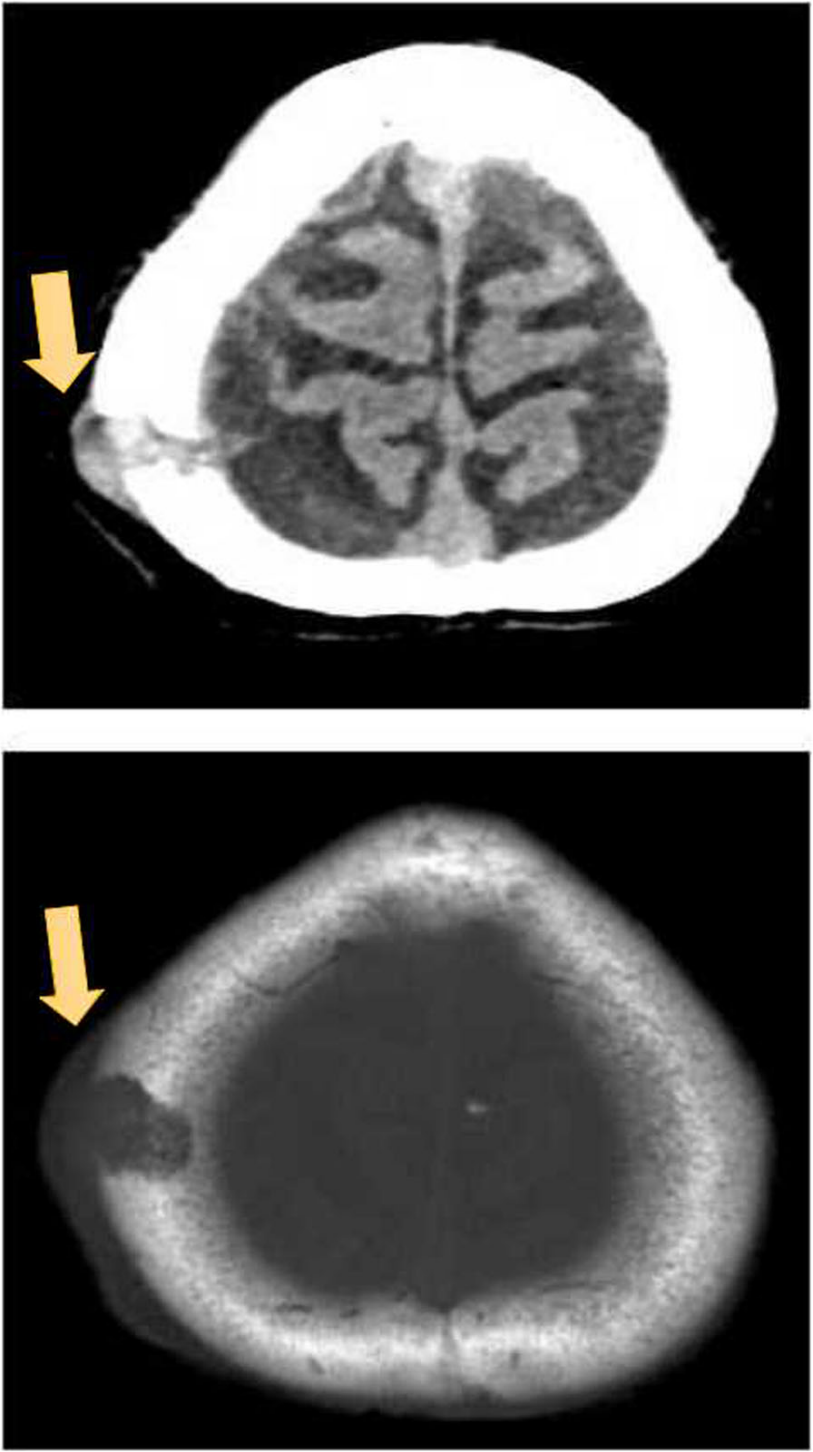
Axial view of non-contrasted computed tomography scan of the brain shows a right parietal bone lytic lesion with soft tissue component (*orange arrows*)
